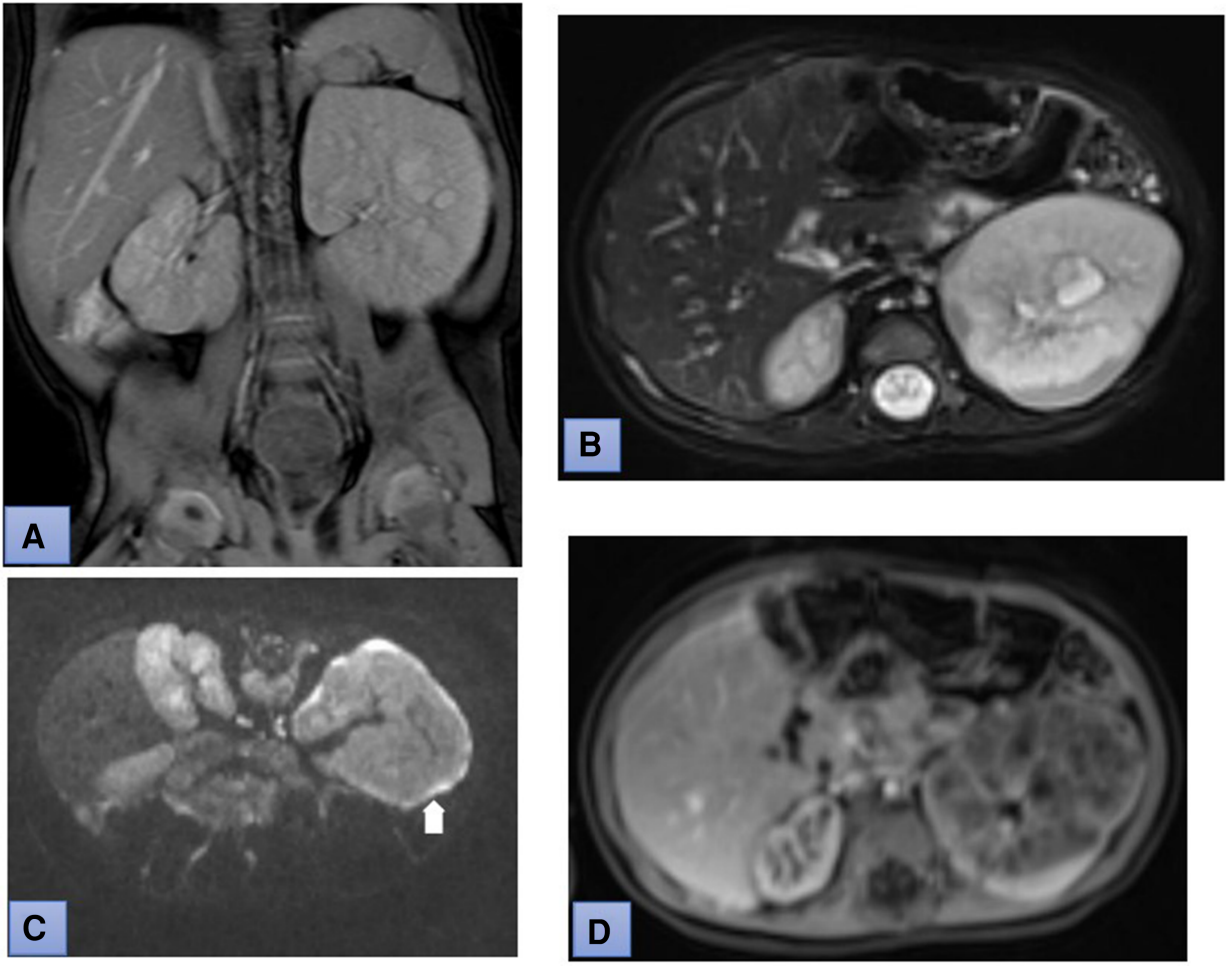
Coronal T2-weighted (A) and fat suppressed T2-weighted (B) MR images demonstrate enlarged both kidneys. Diffusion-weighted MRI (DWI) (*b*-value: 800) and apparent diffusion coefficient (ADC) mapping show uneven thickening of the kidney capsule (white arrow in picture C). Postcontrast T1-weighted MR image demonstrates heterogeneous enhancement of the parenchyma of the left kidney (D).
